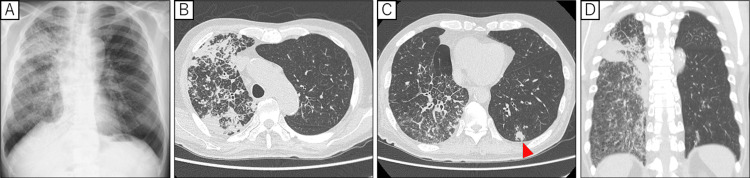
Pre-treatment chest X-ray and CT images showing tumor regression (A) Chest X-ray showed an infiltrative shadow mainly in the right lung field. (B-D) Chest CT showed an infiltrative shadow with thickening of the interlobular septa in the right lung, as well as ground-glass opacity. (C) In addition, a nodule* thought to be an intrapulmonary metastasis was observed in the lower lobe of the left lung (*Intrapulmonary metastasis marked by the red arrowhead).
